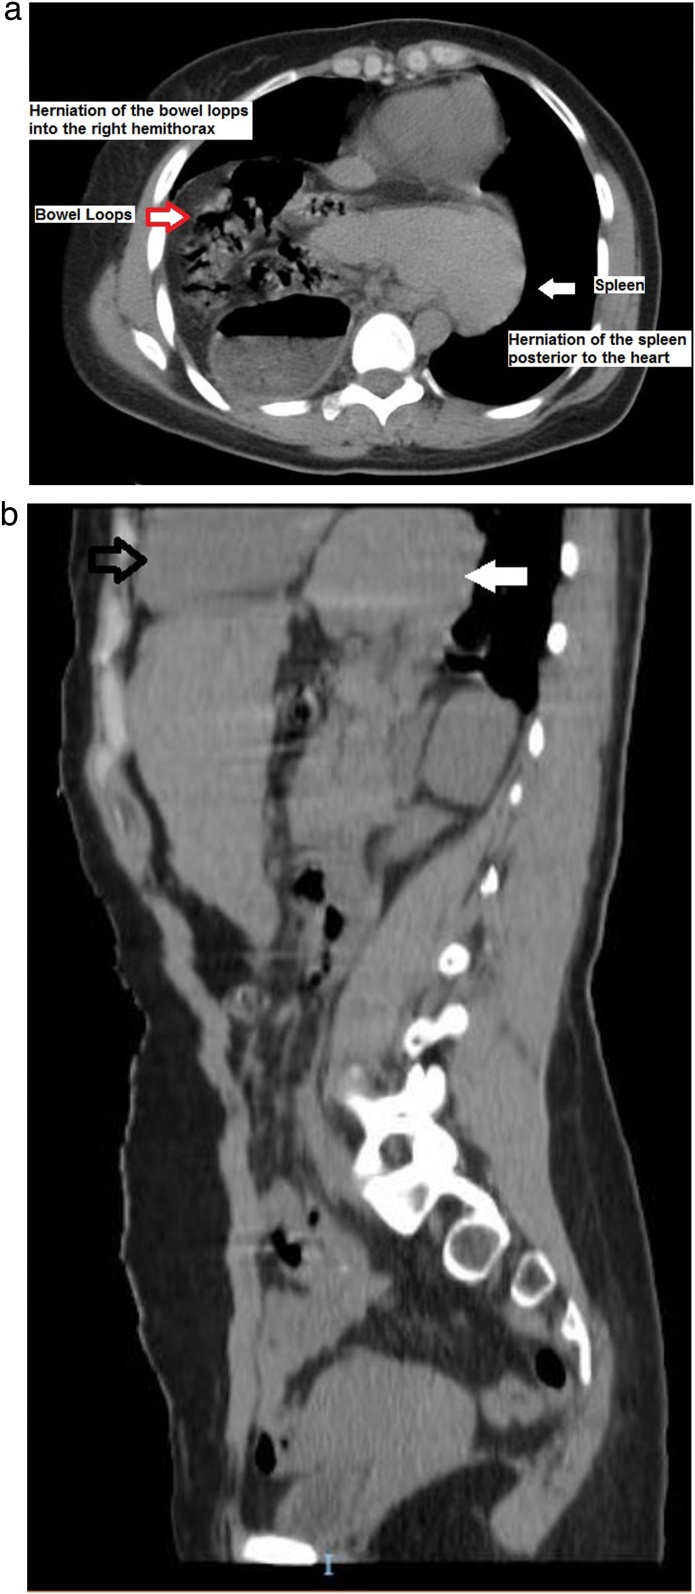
Non-contrast CT-Intrathorasic Extrusion of Spleen, Stomach and Bowels (a); Non-contrast CT-retrocardiac spleen (b).
